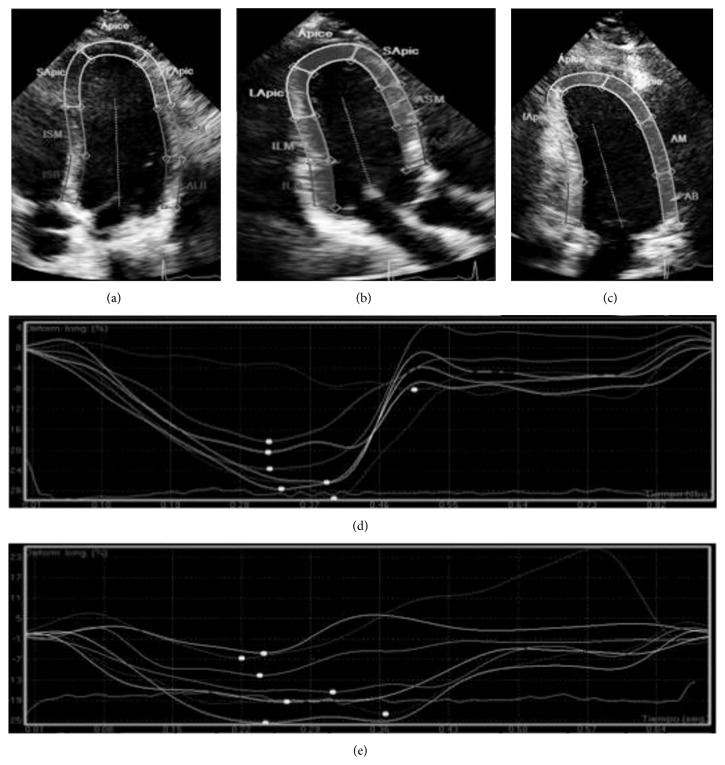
Echocardiographic determination of myocardial longitudinal deformation. Selection of regions of interest for longitudinal strain quantification in four chambers (a), three chambers (b), and two chamber (c) projections. Longitudinal strain curves of a control (d) and hypertensive (e) patient.
